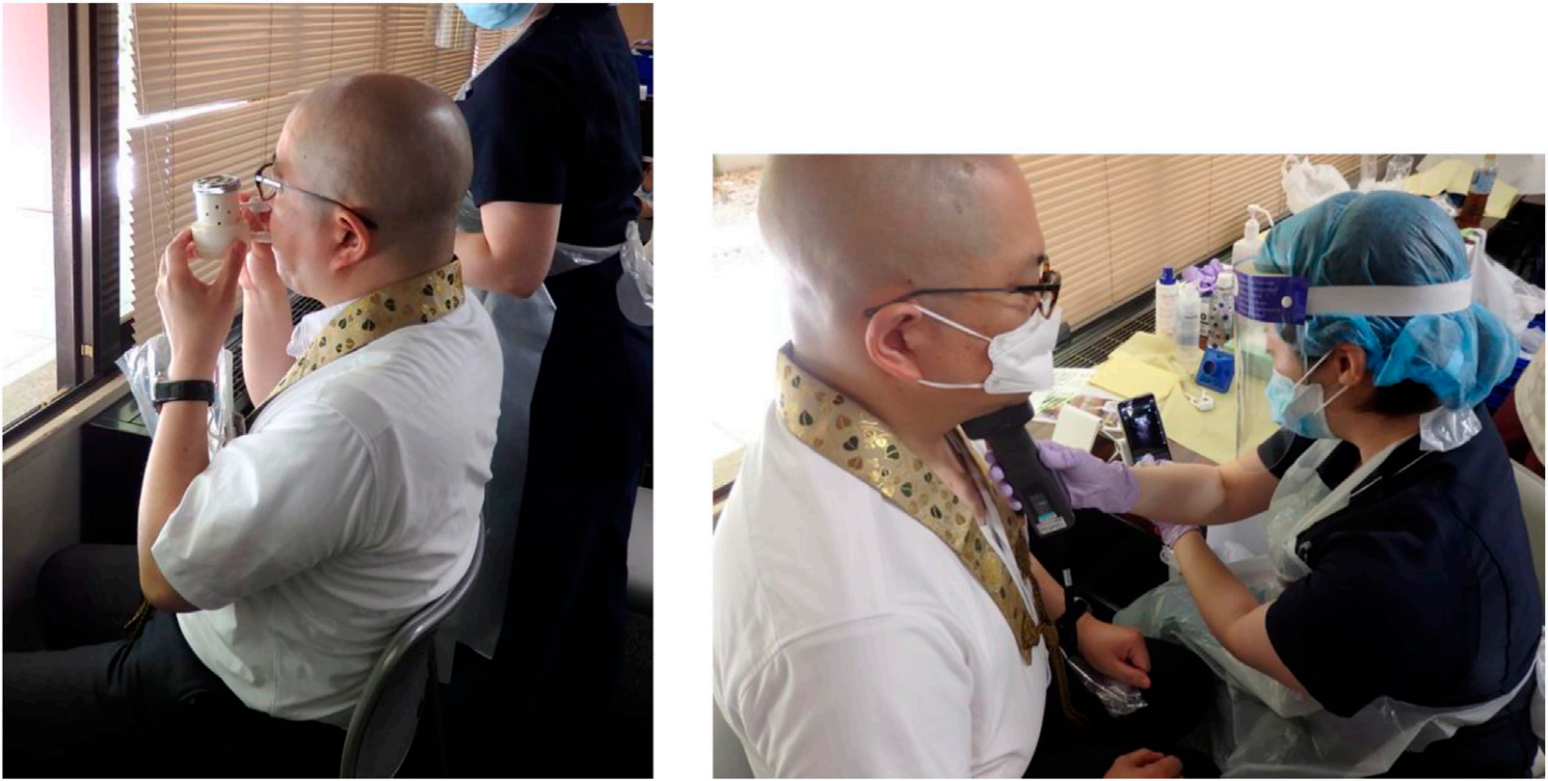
The dentist checked a Buddhist priest’s oral function, respiratory function (left), and hyoid bone displacement by ultrasonic examination (right).
